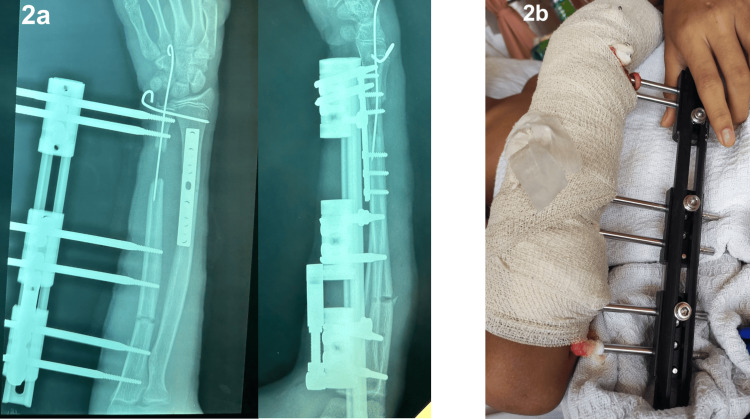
Postoperative radiograph in anteroposterior and lateral views of the right forearm (2a) and postoperative clinical image of the right forearm after the application of the mini limb reconstruction system external fixator for bone transport (2b).
